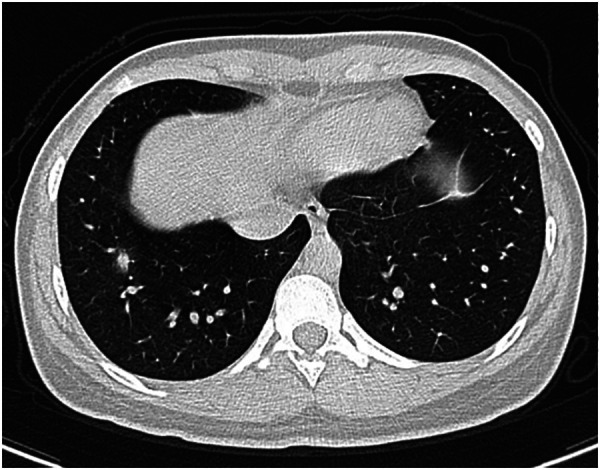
17-year-old girl infected with the Omicron variant of SARS-CoV-2. Unenhanced chest CT scans show pulmonary nodule with peripheral halo sign in the lower lobe of the right lung. CT findings were assigned CT severity score of 1.
